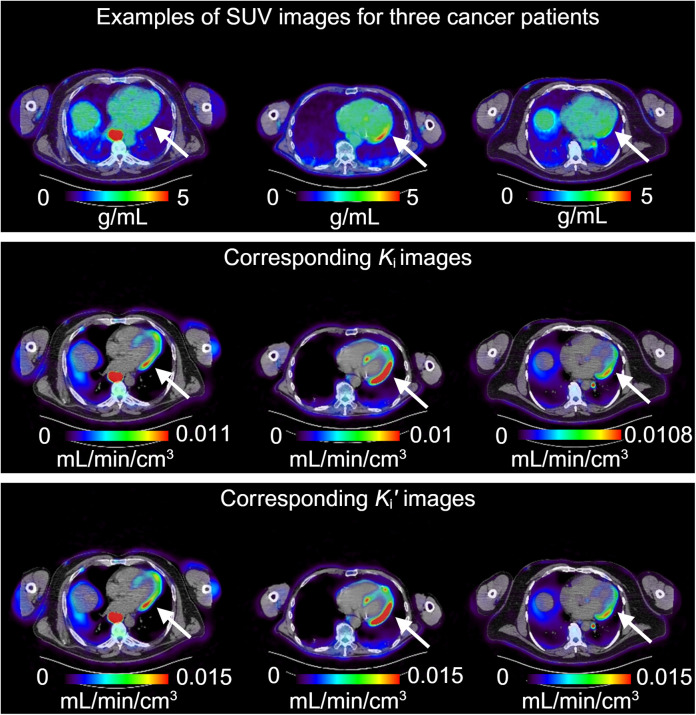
Comparison of parametric images with SUV images to visualize myocardium in 3 cancer patients (genitourinary cancer patients 1, 4, and 7). From top to bottom: SUV, standard Patlak *K_i_*, and RP *K_i_*′ images. All images were superimposed on CT. Arrows point to myocardium regions.
